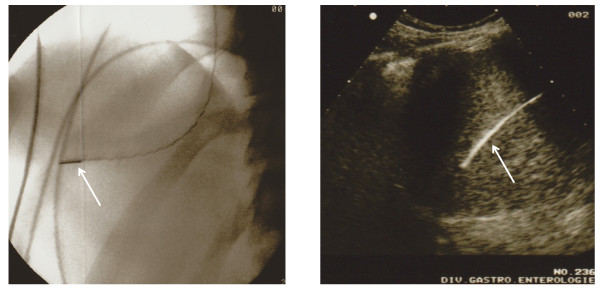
Imaging of the liver by intrahepatic use of an intracardiac echography catheter (ICE). The position of the ICE catheter, here in the right hepatic vein, was assessed by conventional X-ray (left panel, white arrow) and by conventional abdominal ultrasound (right panel, white arrow).
